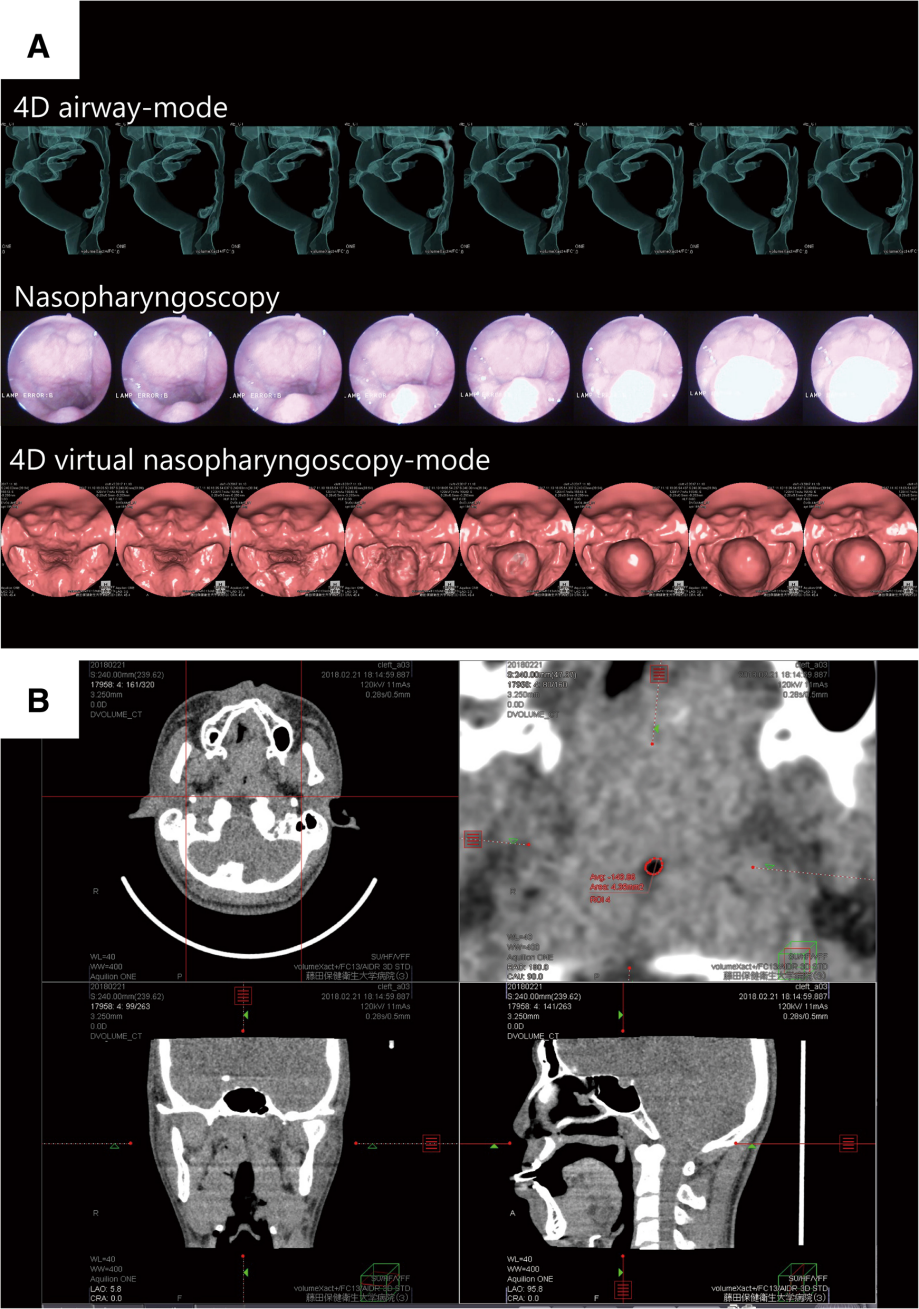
Computed tomography and nasopharyngoscopy images. a Images of the airway in 4D-mode (top), actual images of nasopharyngoscopy (middle), and 4D images of virtual nasopharyngoscopy-mode (bottom) b Multiplanar reconstruction images to calculate the cross-sectional area of velopharyngeal insufficiency
